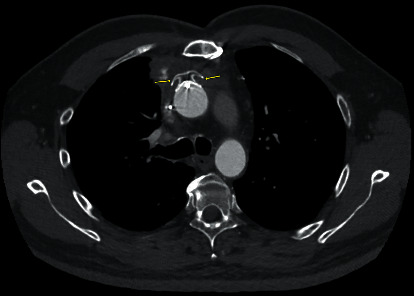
Computed tomography angiography demonstrating no overt contrast flow around a well-sealed ASD Amplatzer occlusion device within the ascending aortic anterior pseudoaneurysm one month following the procedure.
